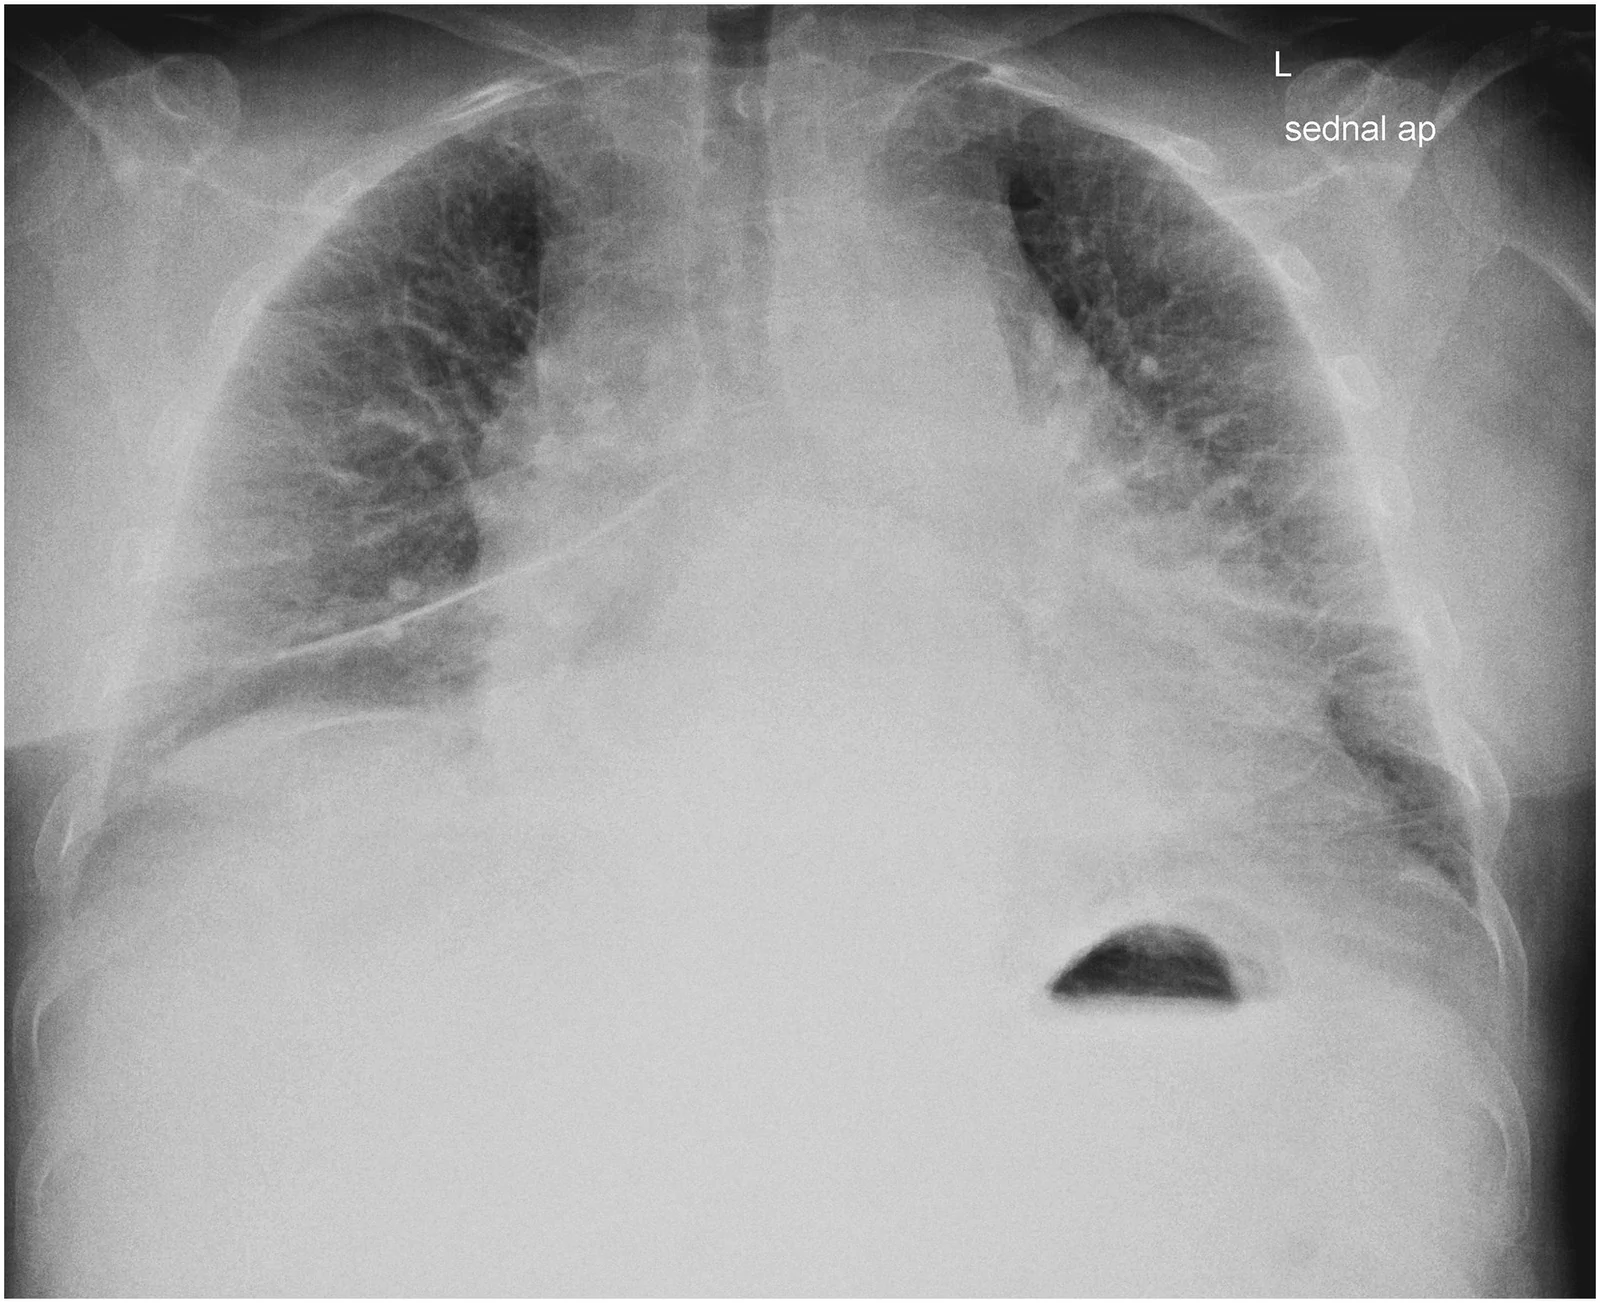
Chest radiography. Chest radiography demonstrated an enlarged cardiac silhouette associated with interstitial pulmonary congestion and bilateral pleural effusions.
